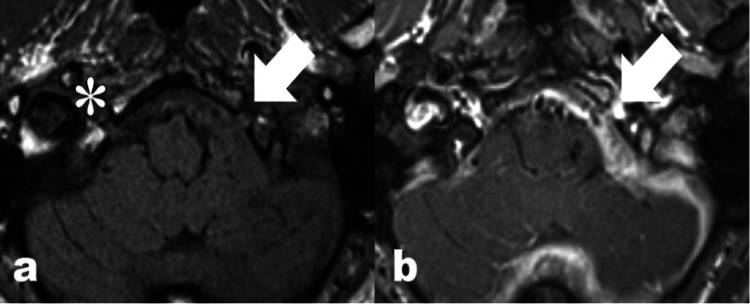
(A): Axial non-contrast small-field-of-view T1 weighted spin echo MRI image of the posterior fossa showing involvement of the left hypoglossal canal by abnormally thickened pachymeninges (arrow). The normally appearing right hypoglossal canal is marked with an asterisk for comparison. (B): Axial post-gadolinium contrast small-field-of-view T1 weighted spin echo MRI imaging at the same location demonstrating avid enhancement of the pachymeningeal thickening (arrow).
